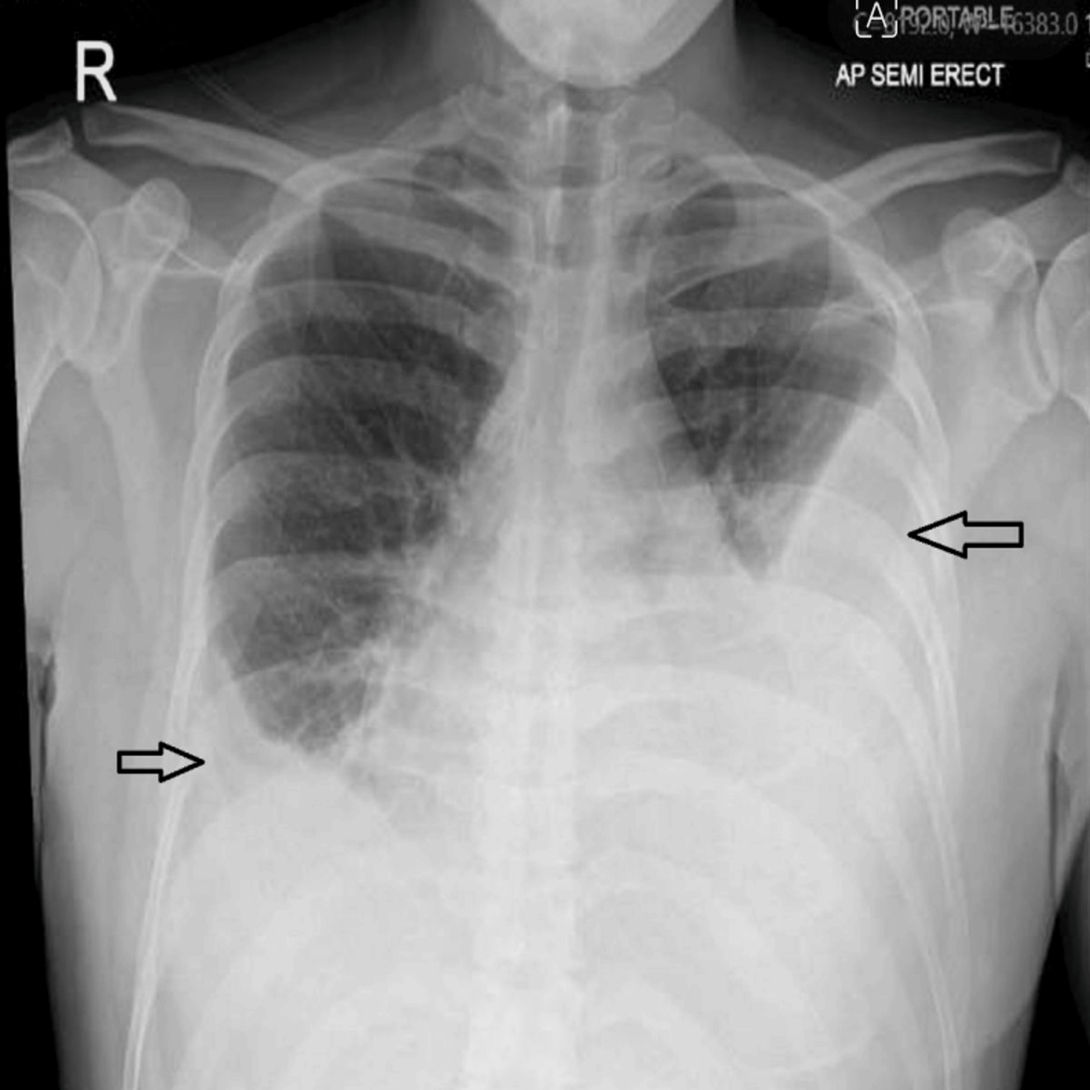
Chest X-ray showing new large left-sided pleural effusion and recurrence of right-sided pleural effusion.
